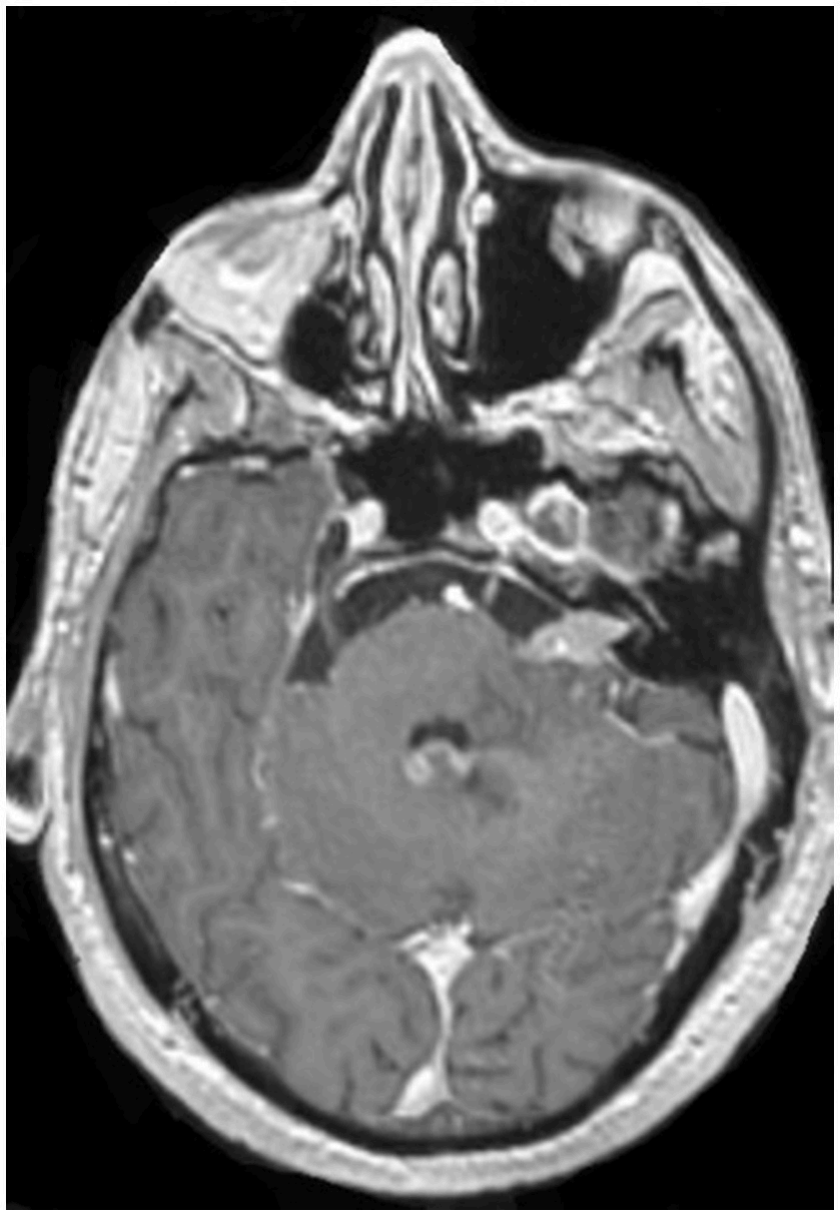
Axial MRI with nodular contrast enhancement in VII cranial nerve and enhancement in fourth ventricle, patient with diagnosis of lung cancer.
